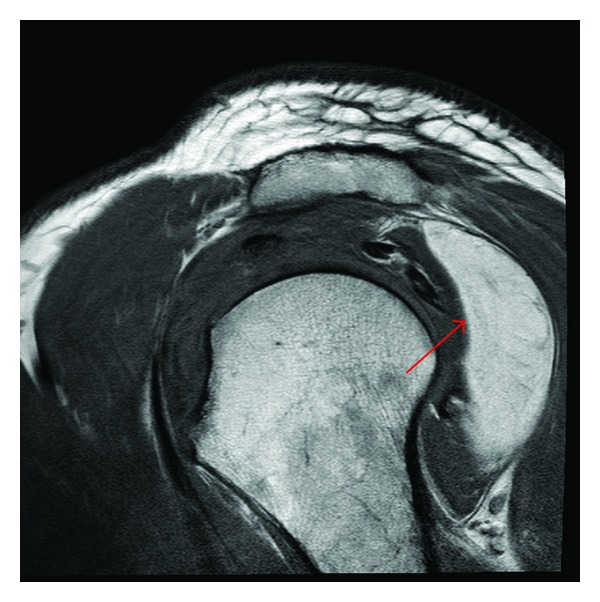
Sagittal T1 TSE magnetic resonance image of right shoulder showing a homogenous lesion (arrow) compatible with an intermuscular lipoma under the posterior aspect of the deltoid, extending to the undersurface of the acromion.
